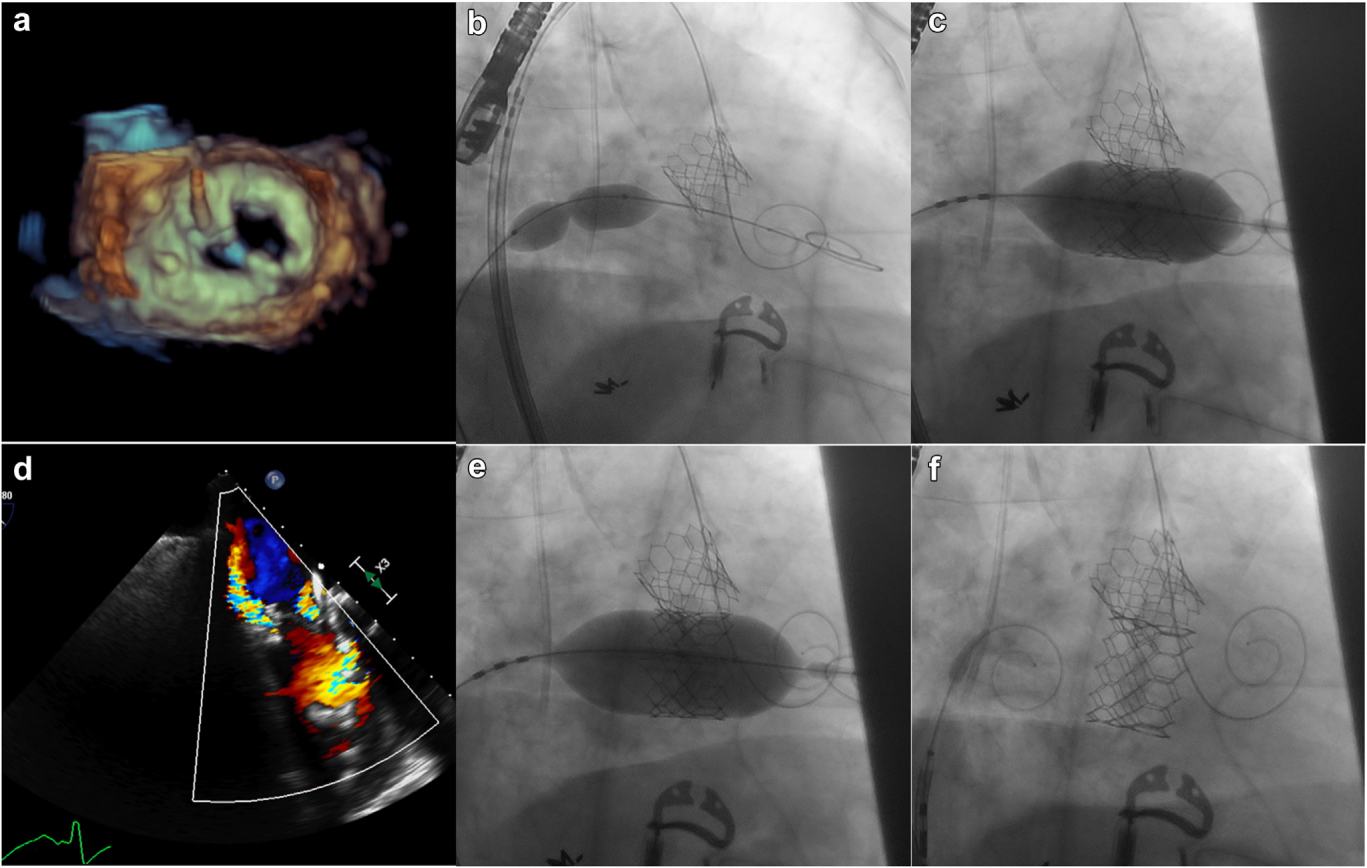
Mitral valve safe crossing, predilation and mitral valve deployment. (a) 3D TEEmitral valve reconstruction to guide the crossing of the mitral valve to get access to the left ventricle (LV). (b) Interatrial septum predilation with a 40 × 16 mm Z-Med balloon. This picture also demonstrates that the Safari^2^ wire (Boston Scientific, Massachusetts, USA) across the aortic valve was voluntarily left in place to be able to intervene (kissing balloon inflation) in case of LVOT obstruction after TMVR. (c) TMVR under rapid pacing through the LV wire. (d). TEE showing a moderate paravalvular leak with no LVOT obstruction. (e) Postdilation of the 26 mm SAPIEN S3 Ultra valve (Edwards Lifesciences, California, USA) with 3 mL extra. (f) Final fluoroscopic result of the simultaneous transcatheter aortic and mitral replacements for native multivalvular heart disease. Abbreviations: LVOT, left ventricular outflow tract; TEE, transesophageal echocardiography, TMVR, transcatheter mitral valve replacement.
